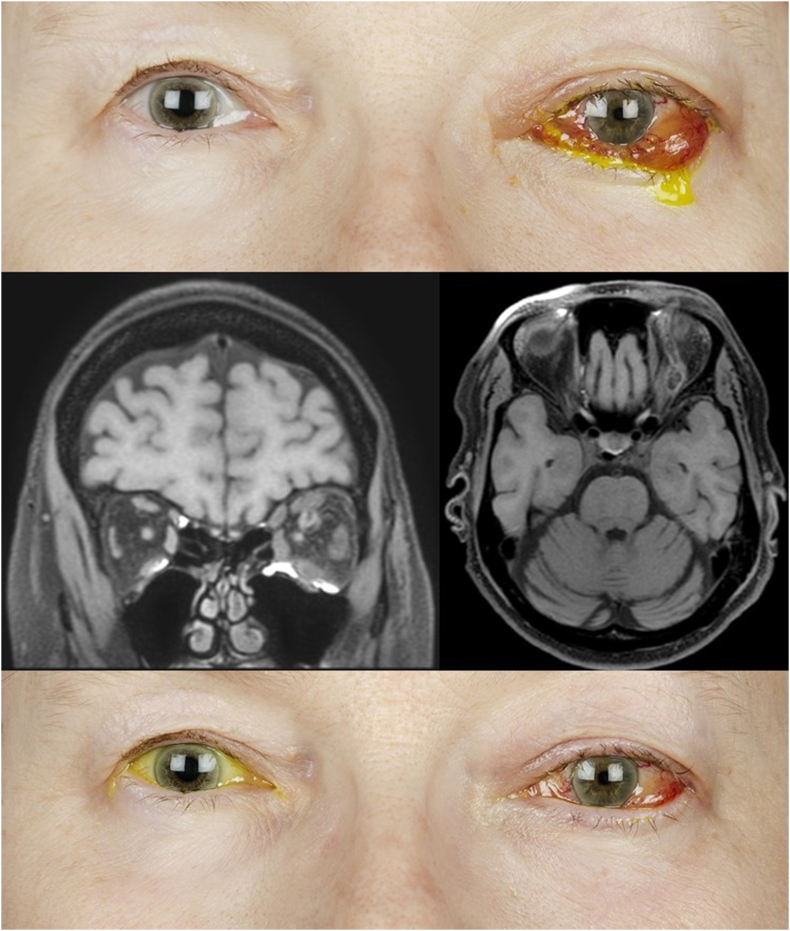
Case 1 – Patient with superior ophthalmic vein thrombosis and cavernous sinus thrombosis. A Photo at initial presentation. B–C Magnetic resonance imaging at initial presentation showing enlarged superior ophthalmic vein, orbital congestion and enlarged ocular muscles. D Photo at follow up three weeks later.
